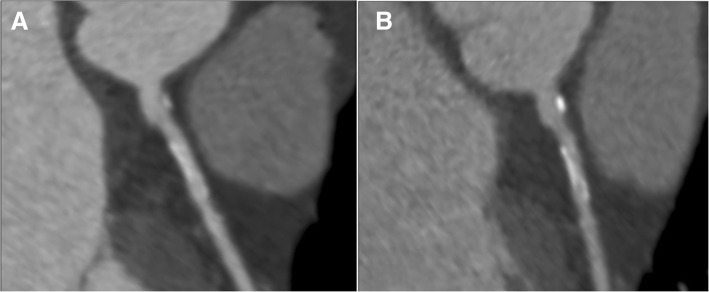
Example of coronary calcification progression at serial coronary computed tomography angiography. Automatically generated curved multiplanar reformation of image data of the left anterior descending coronary artery in an asymptomatic diabetic 60-year-old man performed in May 2011 (a) and in December 2013 (b). There is progression of dense calcification as well as overall plaque volume, while the stenosis of the coronary artery showed no obvious worsening
